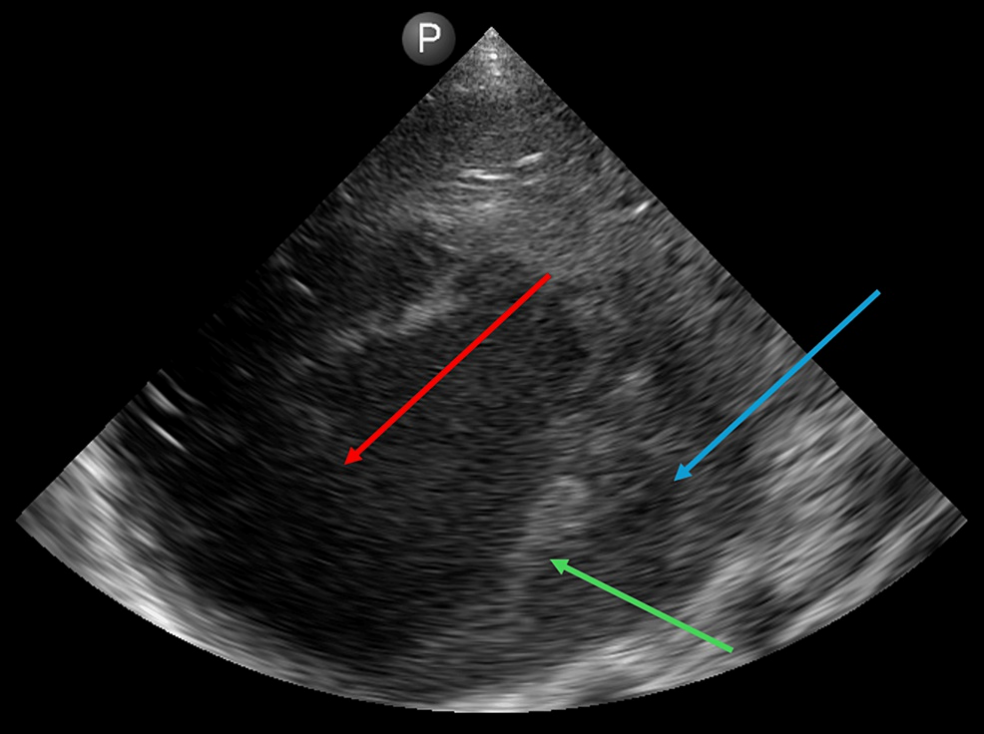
POCUS image of a hypoechoic liver mass with heterogenous echotexture and a hyperechoic rim in the peripheral area of the liver at the time of first drainage. POCUS: point-of-care ultrasound. The blue arrow indicates the liver, the red arrow indicates the abscess, and the green arrow indicates the hyperechoic rim.
